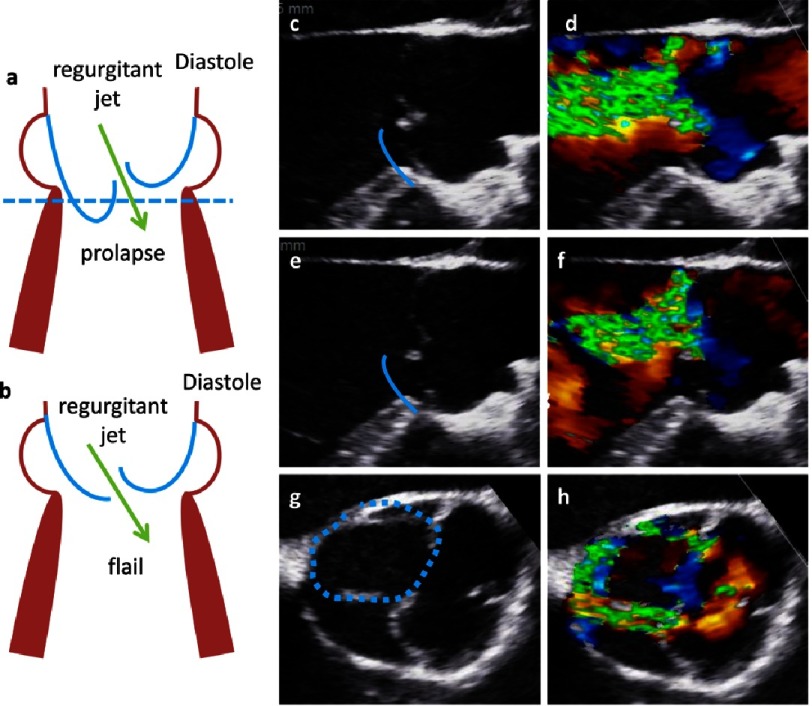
In the scheme a prolapse (a) and a flail (b) are shown in long axis views. On the right side native (c) and color-coded 2D transesophageal long axis view is shown documenting the free margin of the RCC in the LVOT during diastole and the regurgitant jet. Below oblique corresponding views are shown. The course of the RCC and the prolapse are labeled by the blue lines in the native 2D transesophageal images. In (g), (h) color coded 2D transesophageal short axis views show the prolaps by the colored contour displayed by the blue dotted line.
